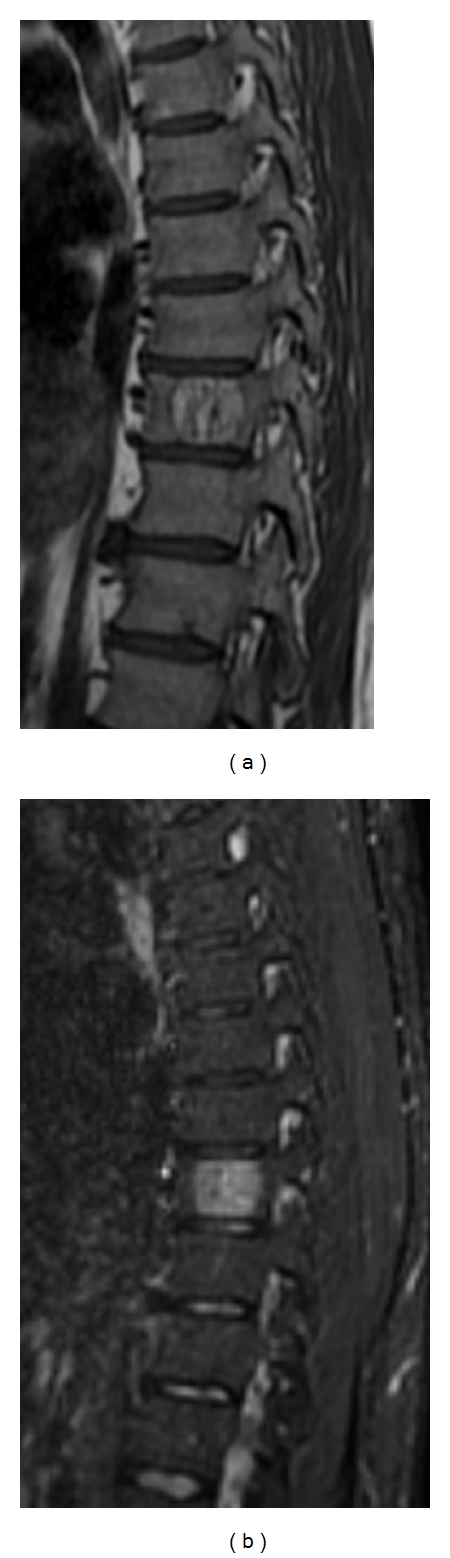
Sagittal T1-weighted (a) and STIR (b) MR images depict a well-circumscribed lesion in a mid thoracic vertebral body. The hyperintense signal and linear hypointensities, which correspond to thickened trabeculae, are characteristic of a benign hemangioma.
